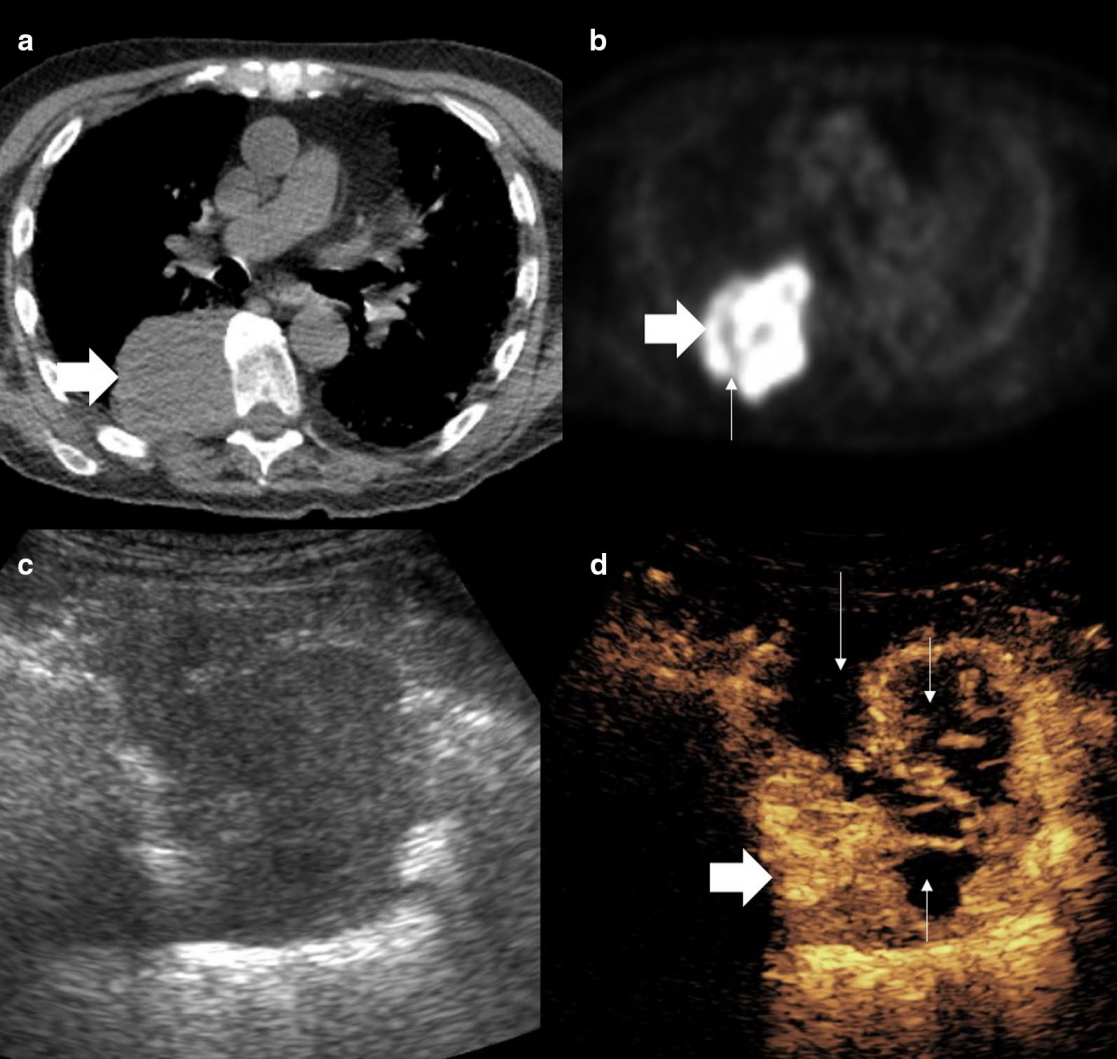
Patient with squamous cell carcinoma. a CT examination shows a solid appearing lung lesion (arrow). b PET-CT suggests small areas of necrosis (thin arrow) within the avid lesion (arrow). c, d Simultaneous B mode and CEUS images show the hyperenhancing lesion (arrow) with better appreciation of the complexity and volume of necrosis (thin arrows) indicating a deep eccentric portion required targeting for biopsy (arrow)
